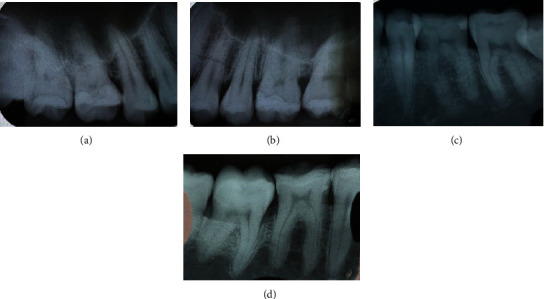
Periapical radiographs of (a) teeth 16 and 17, (b) teeth 26 and 27, (c) tooth 36, and (d) tooth 46 at 21-year SRCT follow-up examination.
